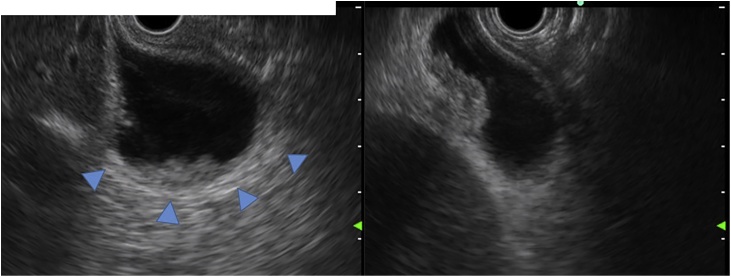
Endoscopic Ultrasonography Imaging. (For interpretation of the references to colour in this figure legend, the reader is referred to the web version of this article.) The continuity of the 3^rd^ layer was confirmed (blue triangle). The invasion depth was assumed to be subserosa.
